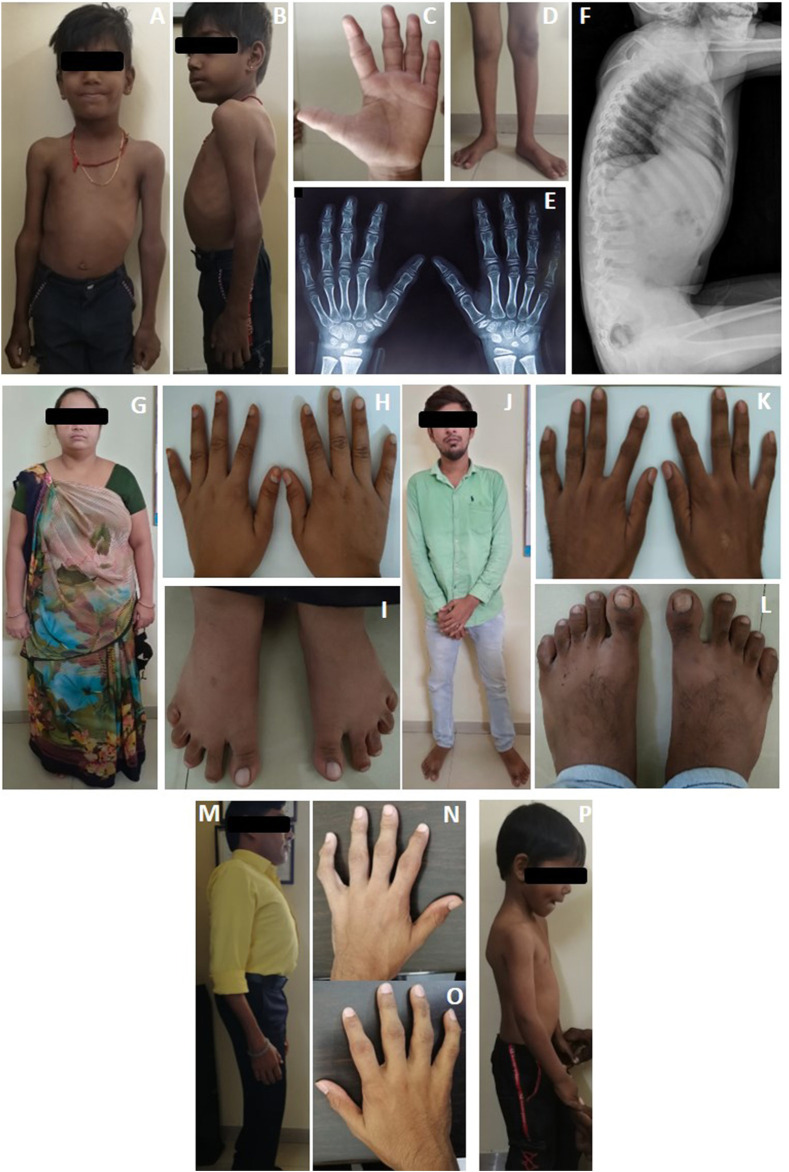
Clinical photographs and radiographs of proband's (A–F) case 1, (G–H) case 1’s mother, (J–L) case 1’s father, (M–O) case 2, and (P) Case 3. Case 1 is observed with (A) chest deformity and enlarged elbow joints; (B) scoliosis; (C) deformities of interphalangeal joints; (D) knocked knees; (E) radiograph anterior-posterior view, showing osteopenia; (F) anterior beaking of vertebrae. (G–I) Clinical picture of mother (carrier) of case 1; (J–L) clinical picture of father (carrier) of case 1. Case 2 is observed with (M) scoliosis and (N,O)clinodactyly with deformities of interphalangeal joints. Case 3 is observed with (P) mild scoliosis.
